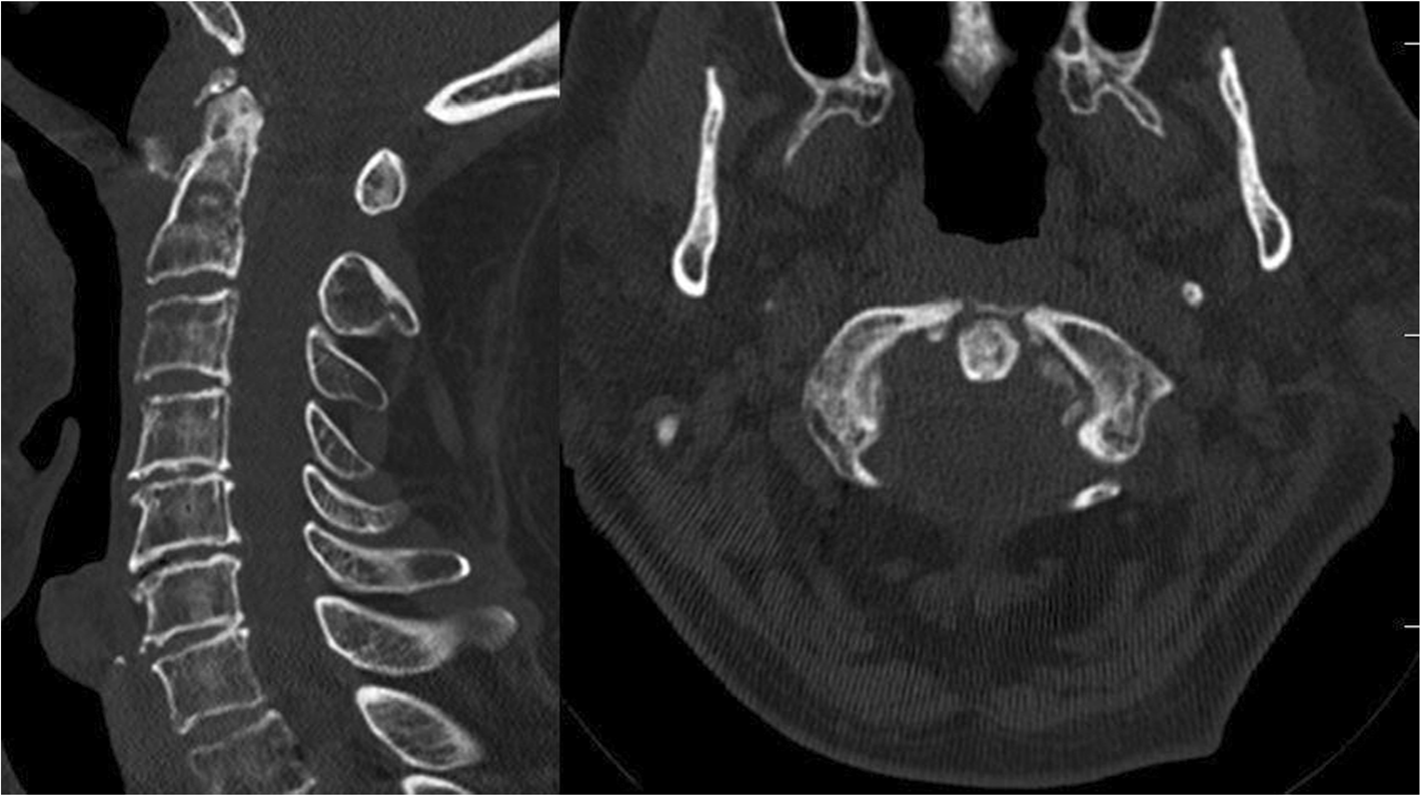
2-month follow-up mid-sagittal and axial CT slices demonstrating a maintained reduction of the anterior arch of C1
